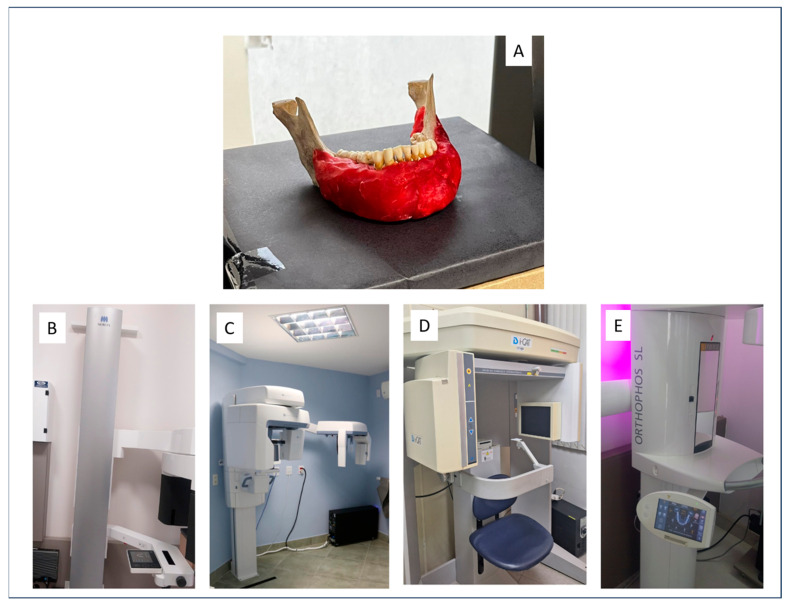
CBCT acquisition setup and systems used: (A) mandible positioned on the standardized rectangular base; (B) Veraview^®^ X800 (J. Morita MFG. Corp., Tokyo, Japan); (C) OP300 Pro^®^ (Instrumentarium Dental, Tuusula, Finland); (D) I-CAT Next Generation^®^ (Imaging Sciences International, Hatfield, PA, USA); (E) Orthophos XG 5 (Dentsply Sirona, Bensheim, Germany).
